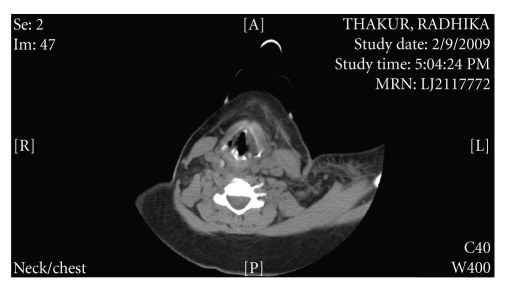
CT Neck showing the abscess.
